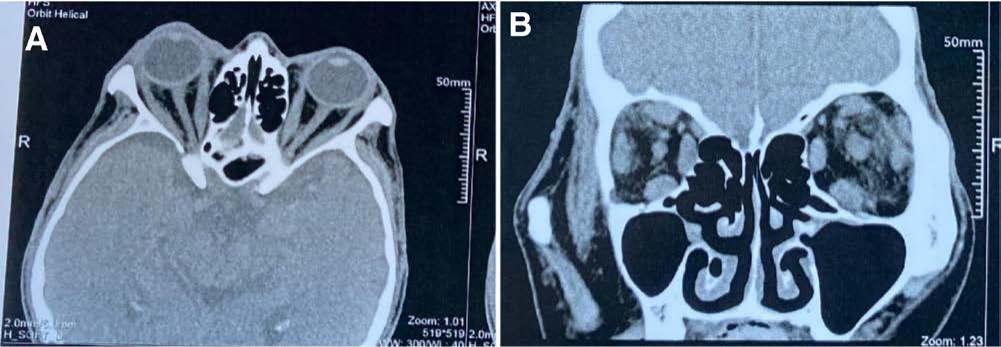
CT scan showing bilateral exophthalmos, bilateral optic nerves, and bilateral thickening of the superior rectus, inferior rectus, medial rectus, and lateral rectus muscles. (A) horizontal position, (B) coronal position.
